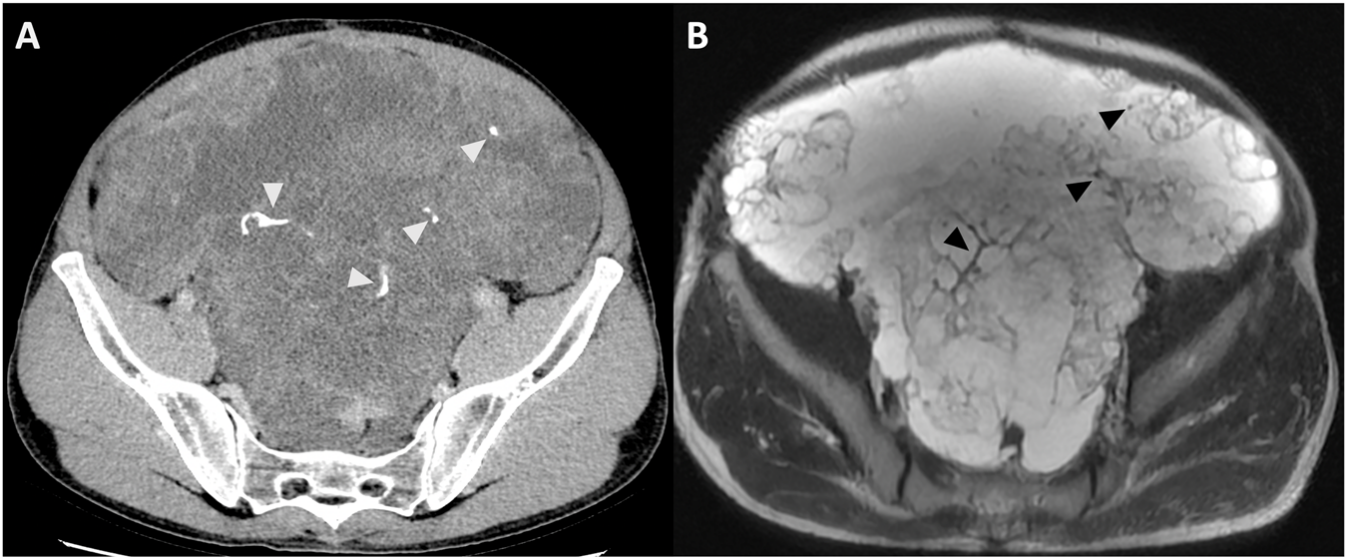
Mucinous colon cancer with peritoneal implants in a 57-year-old man. CT delayed phase (A) and MRI T2w (B) showed calcifications (arrowheads) within the mucinous implants
